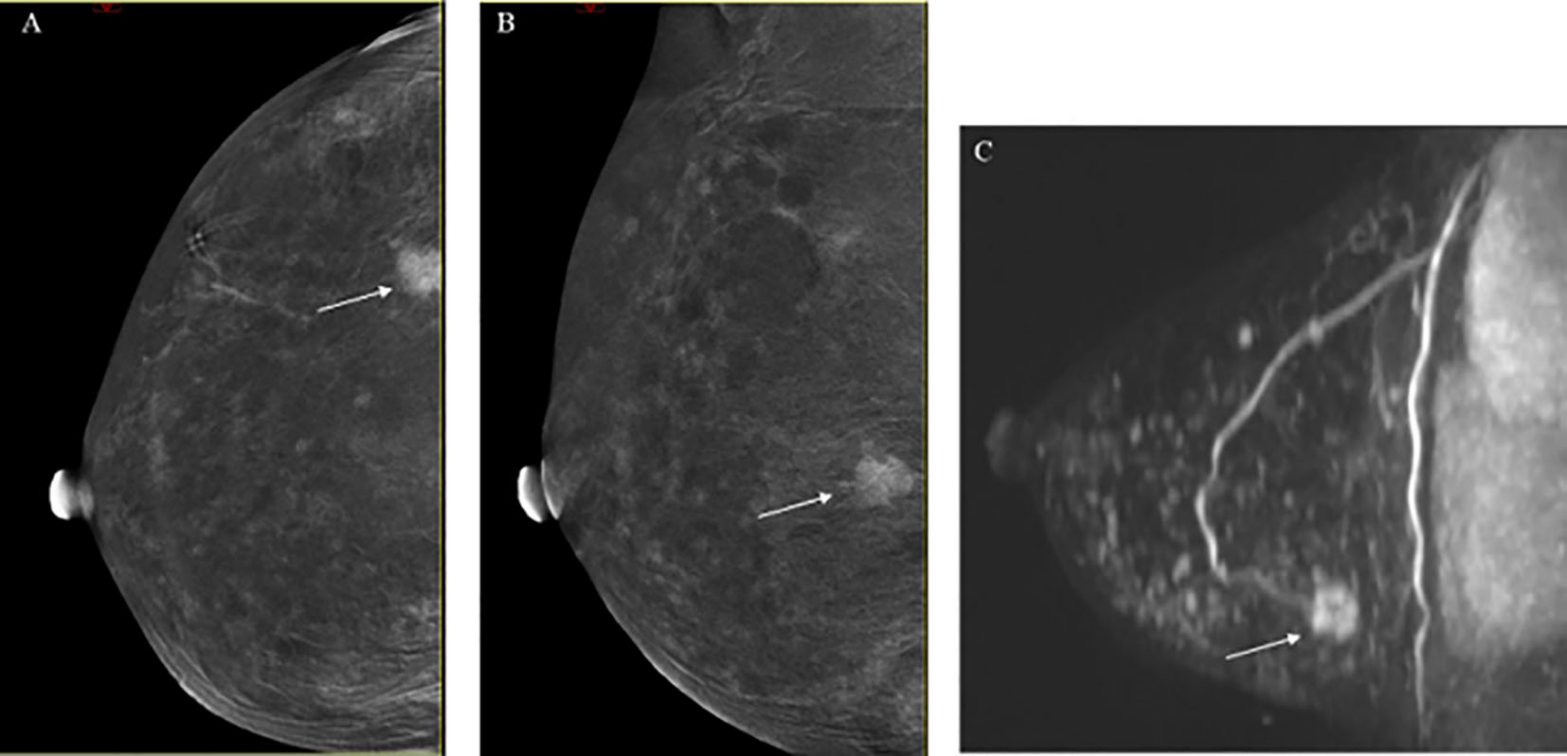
Correct diagnosis of malignant cases by CEM and MRI imaging. (A) CEM CC view (B) CEM MLO view: A slightly high-density mass (indicated by the white arrow) is visible in the lower outer posterior part of the right breast. The mass is lobulated with spiculated borders and shows marked enhancement. This corresponds to (C) the MRI MIP image, where a relatively large blood vessel is observed entering the lesion. The pathological result is invasive ductal carcinoma.
